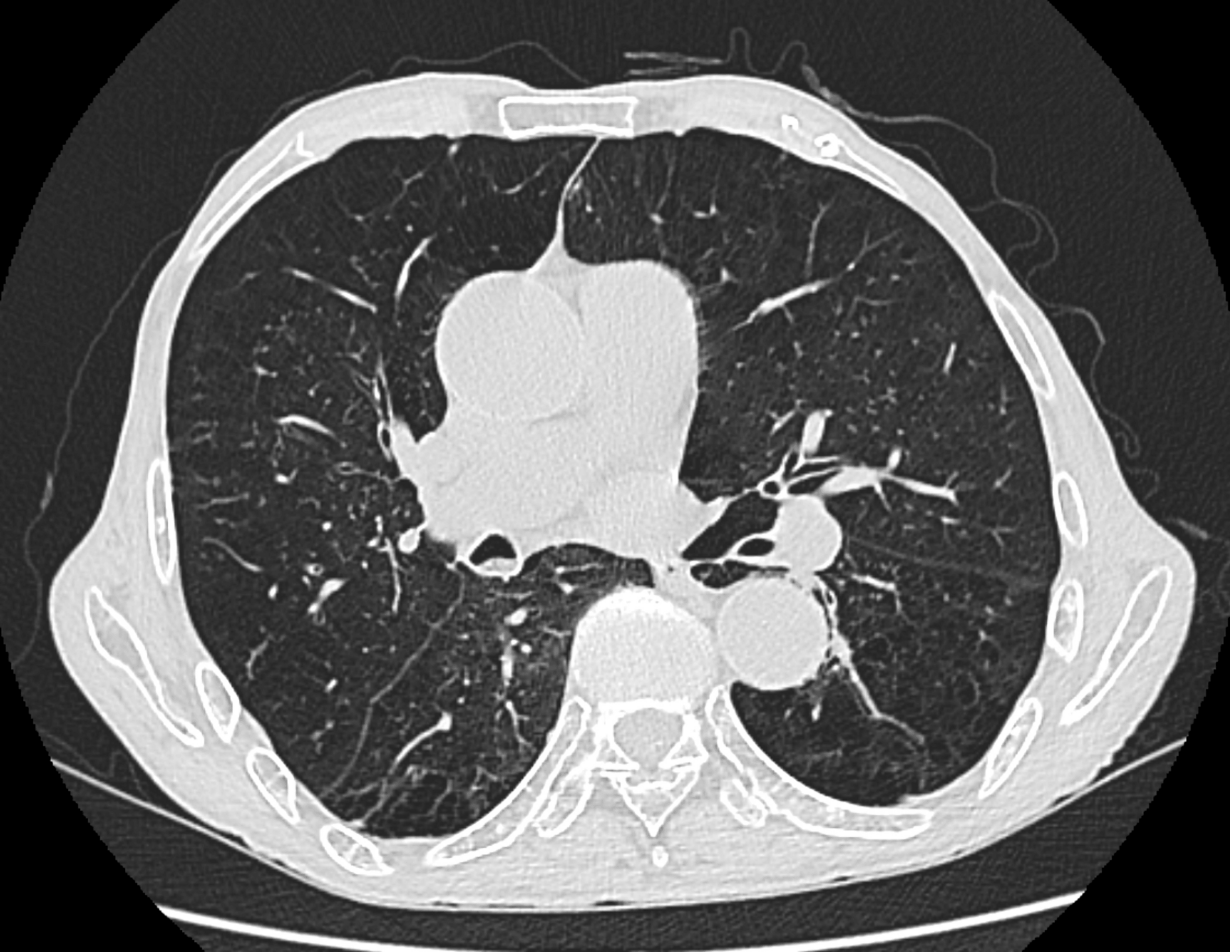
CT of the chest indicating chronic bronchitis with emphysematous changes Chronic pulmonary pathology, excluding acute pulmonary infection.
